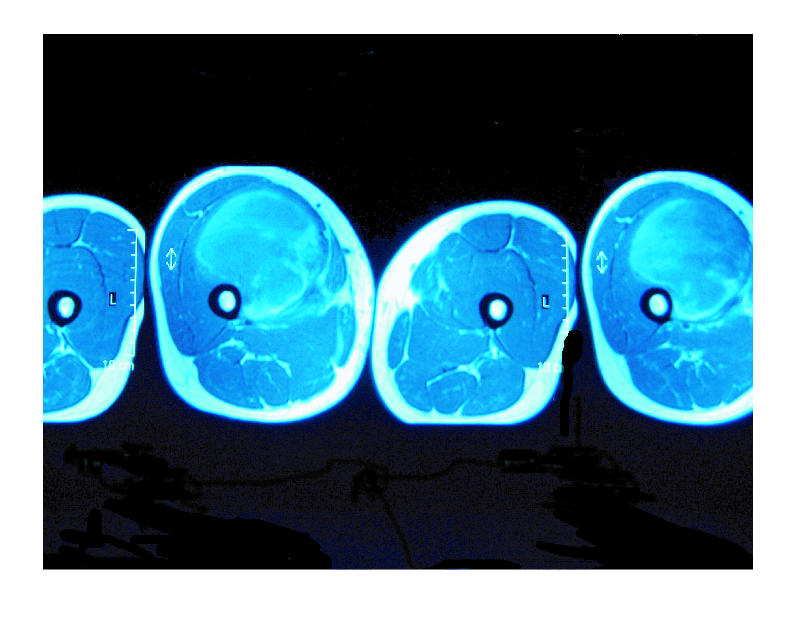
T1-weighted axial spin-echo pulse sequence. Huge mass seen in the right anterior thigh. Peripheral high signal is in keeping with methaemoglobin.
